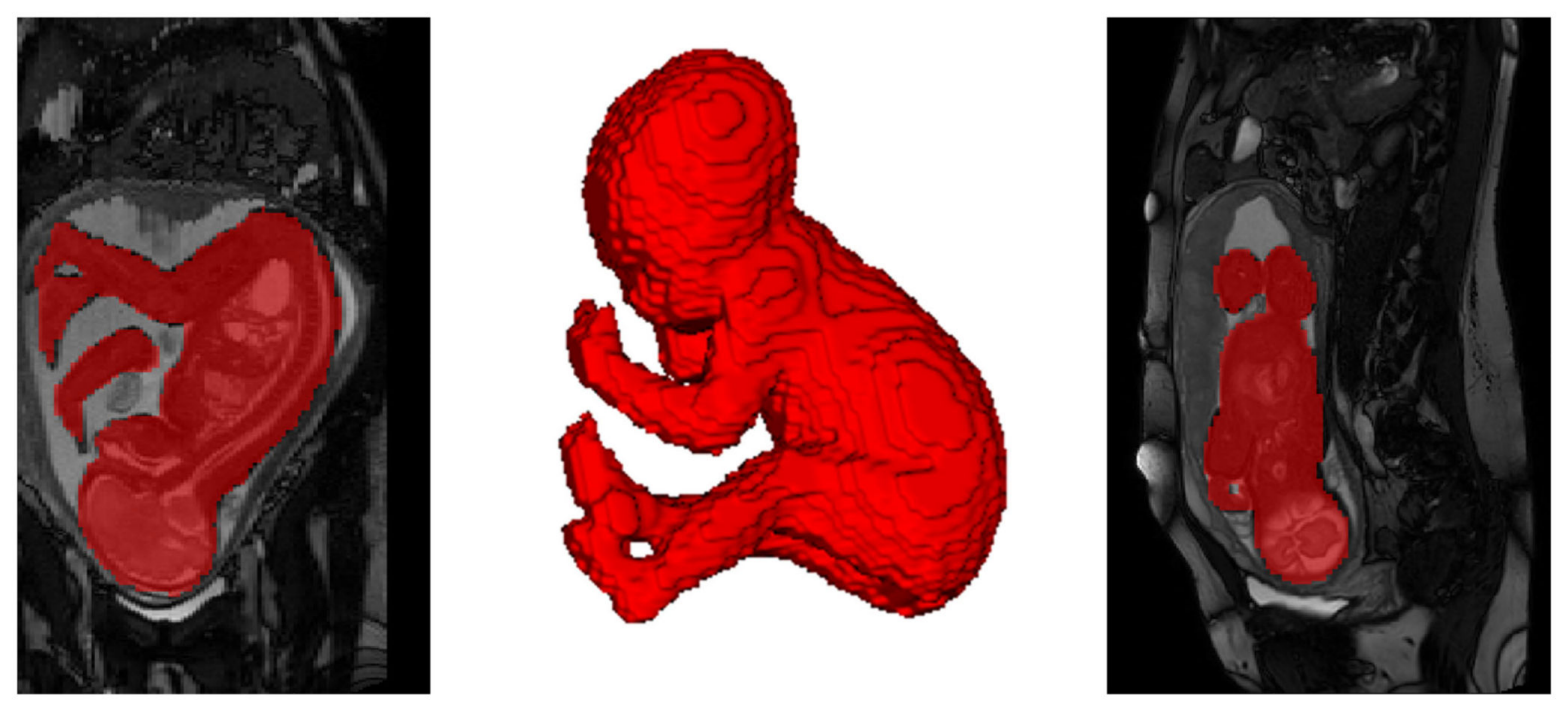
Automatically generated whole-fetus segmentations, allowing estimation of total fetal body volumes, from T2-weighted balanced steady-state free precession (bSSFP) sequences. Coronal bSSFP + segmentation (left), 3D fetal body rendering (middle), Sagittal bSSFP + segmentation (right).
